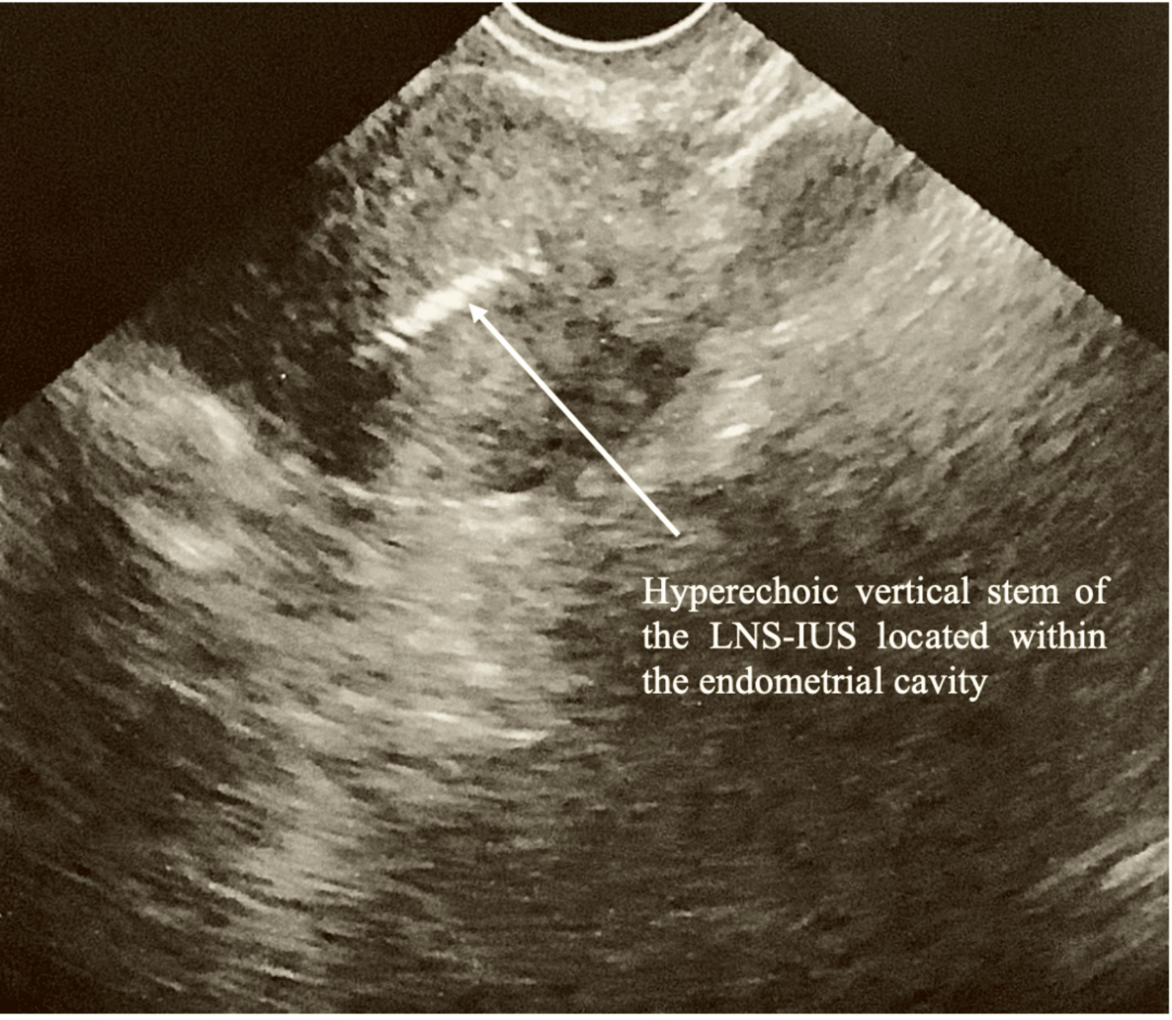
Two-dimensional (2D) transvaginal sagittal ultrasound confirming the presence of the vertical LNG-IUS stem within the endometrial cavity upon insertion. LNG-IUS, levonorgestrel-releasing intrauterine system
